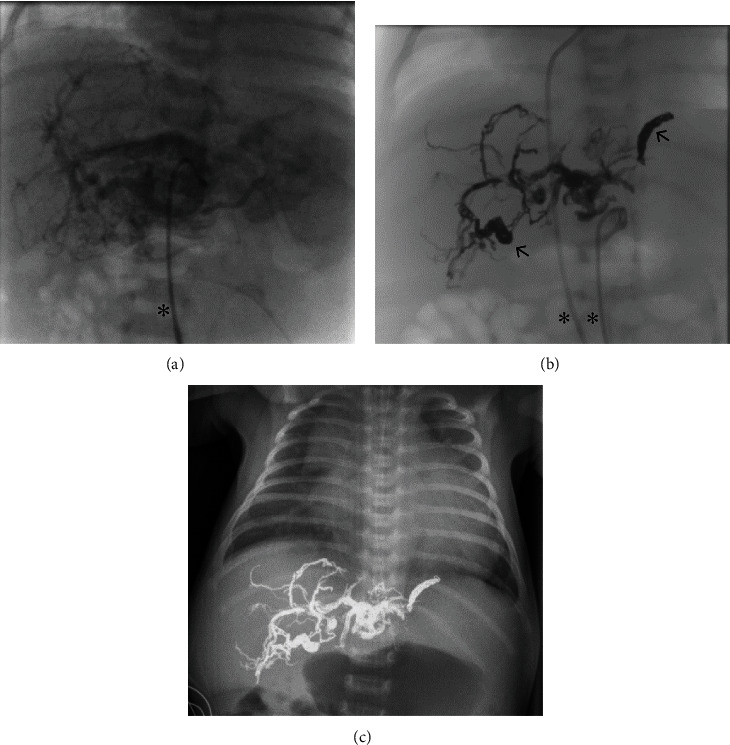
Imaging of the hepatic AVM in patient 4. (a) Angiographical imaging after injection of Solutrast 300® into the feeding arteries via transarterial catheter (asterisk). (b) Angiographical imaging after injection of radiopaque Onyx® and Concerto Helix©-coils (arrows; two transvascular catheters are marked by asterisks). (c) Radiographic imaging (X-ray) of the thorax and upper abdomen after the last embolization, still existing extensive cardiomegaly.
